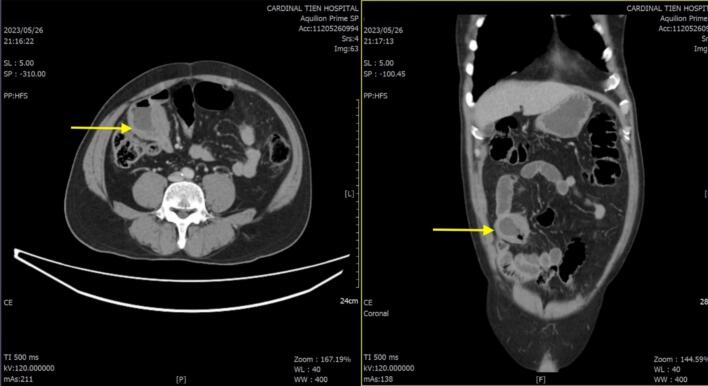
Computed tomogram (CT) of the abdomen with contrast revealing focal dilatation and annular wall thickening of the distal ileu.
